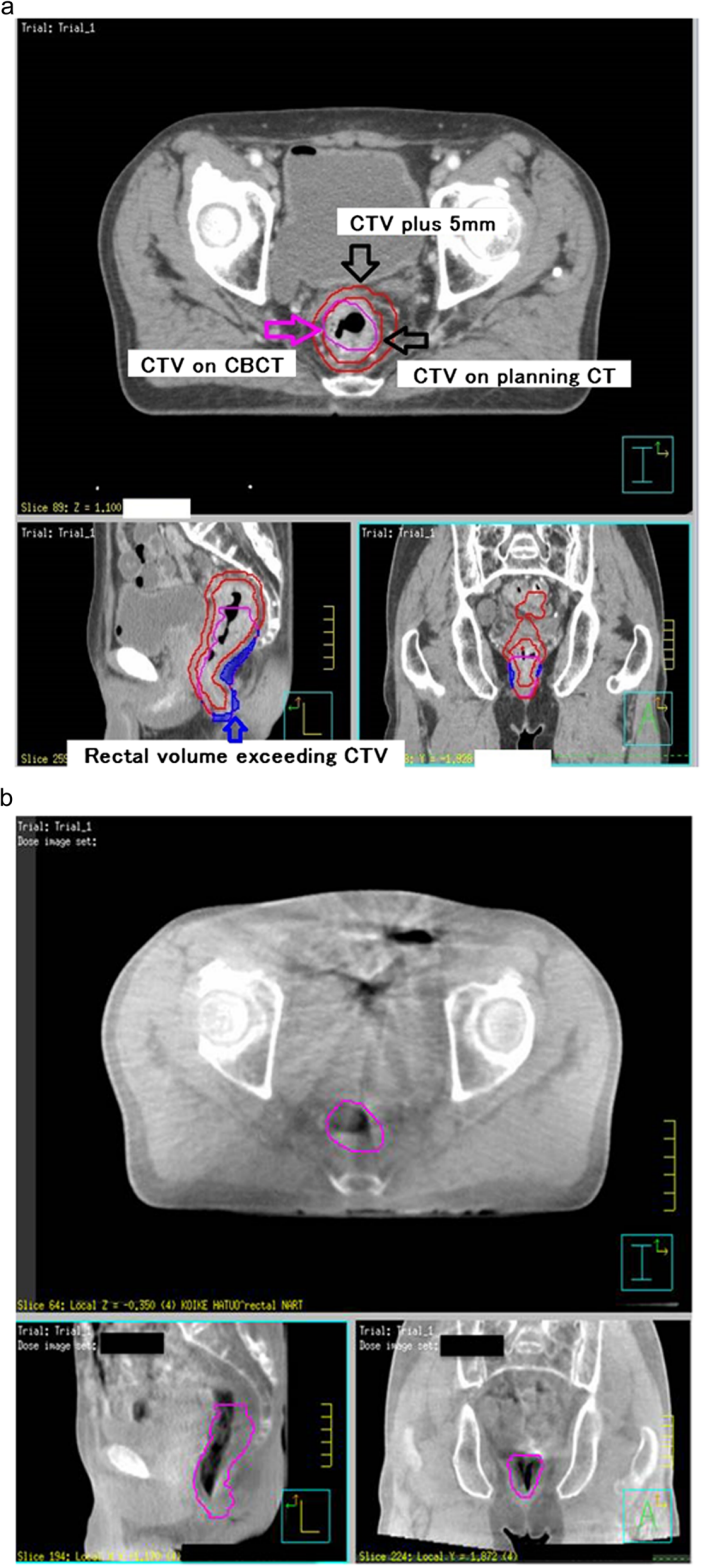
Contouring of the rectum on planning CT (a) and CBCT on 7 days (b). In Figure 1a, the inner red line is CTV (= rectal volume) countured on planning CT, the outside red line is CTV plus 5 mm margin, the pink line is CTV on CBCT on 7 days, and the blue mesh is CTV on CBCT exceeding CTV on planning CT plus 5 mm.
